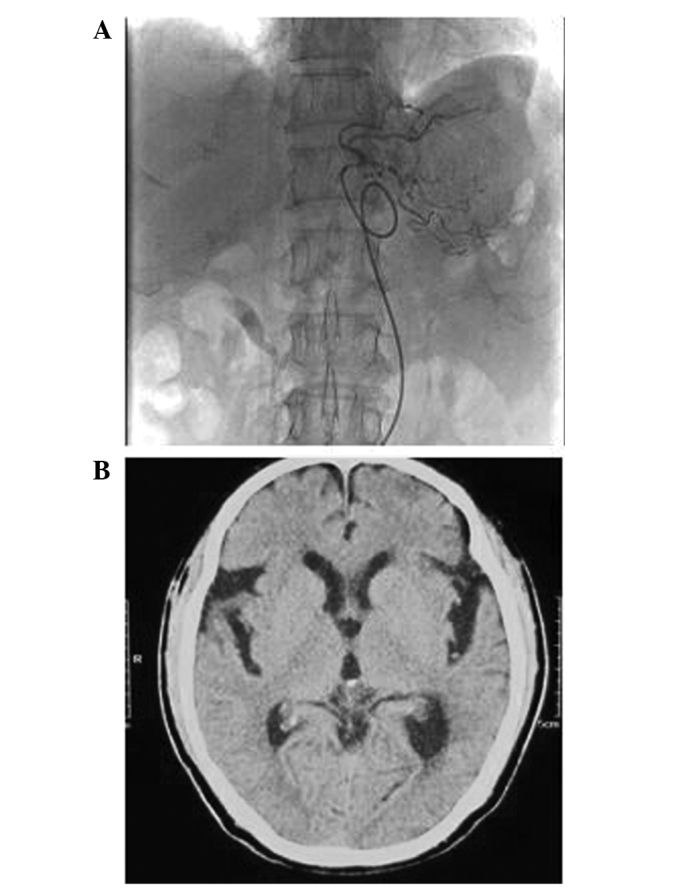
(A) Angiography reveals blood supply to the primary lesion via the left gastric artery. (B) Axial CT scan shows that the brain metastasis in the left temporal lobe has disappeared following four cycles of intra-arterial infusion chemotherapy. CT, computed tomography.
